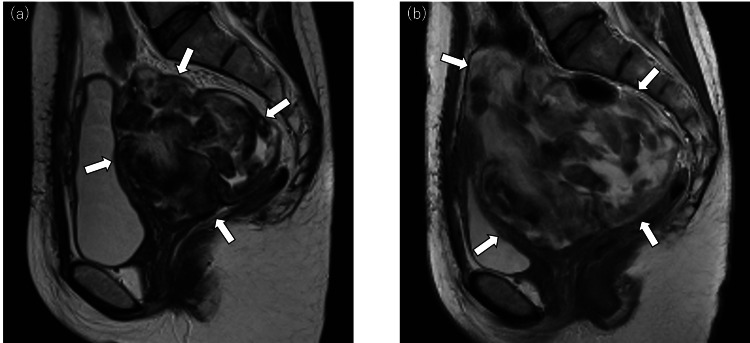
MRI findings at our hospital (white arrows delineate the margins of the tumor.) (a) MRI remained that the residual tumor grew to 10cm. (b) MRI revealed that the tumor grew to 13cm at the time of visiting our hospital.
